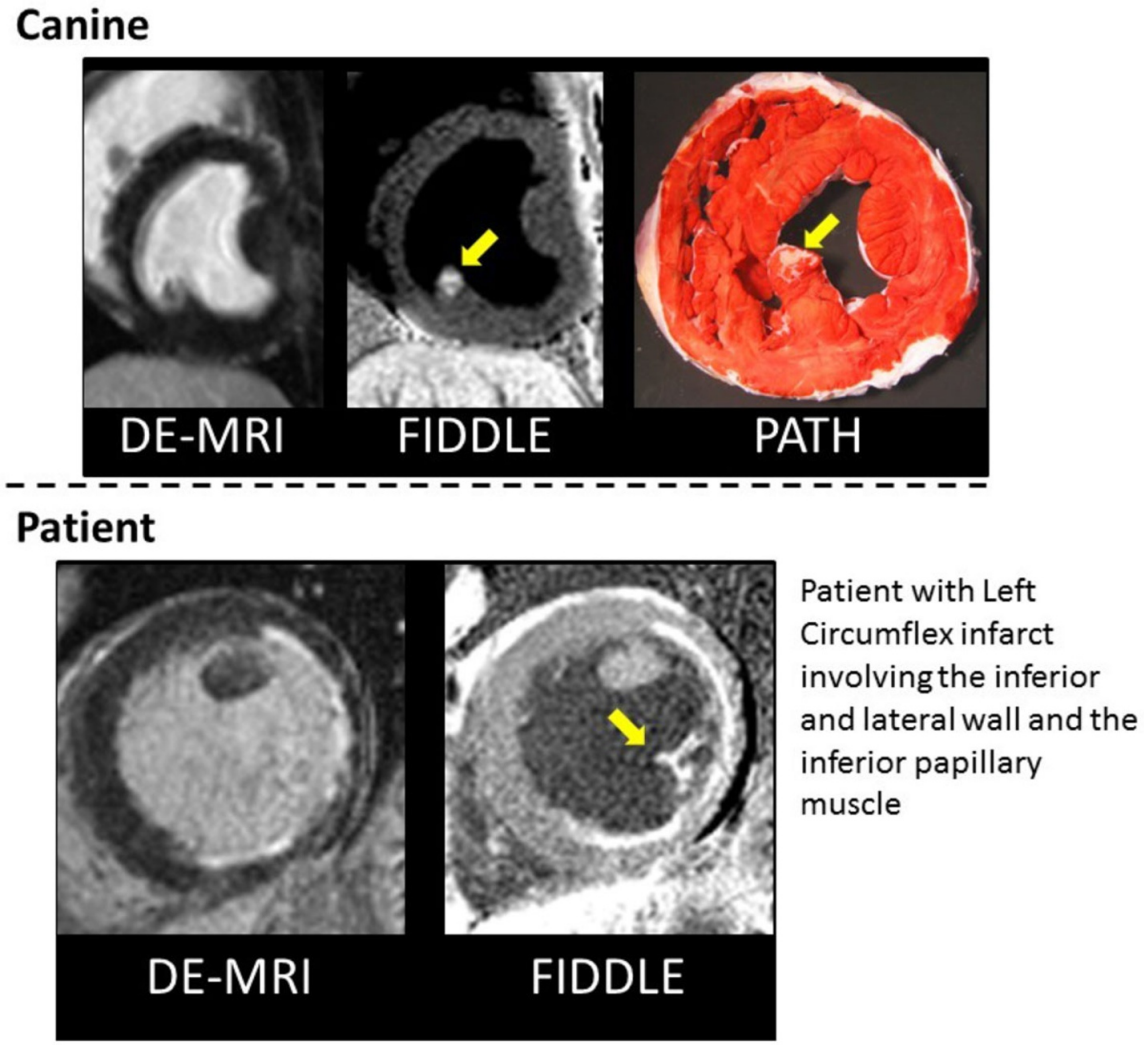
DE-MRI (left), FIDDLE (center), and TTC (right) showing myocardial infarction of only the inferior paillary muscle (arrows). Bottom: patient example of left circumflex coronary artery infarction showng subendocardial hyperenhancement and papillary infarction
